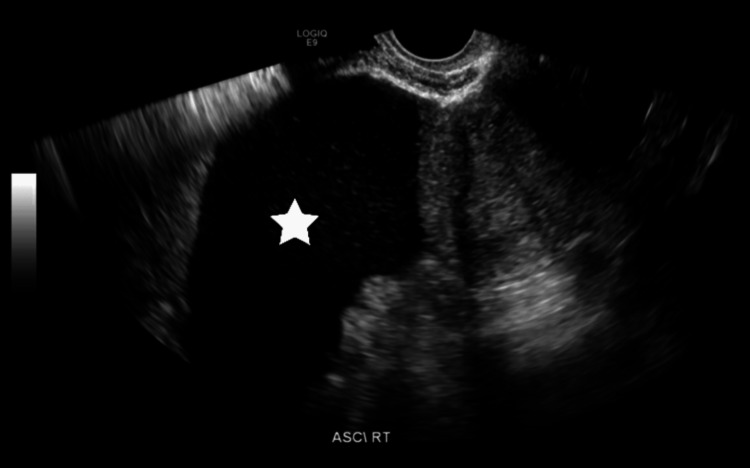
An ultrasound of the abdomen showing moderate free intra-abdominal fluid on the right lower side.
